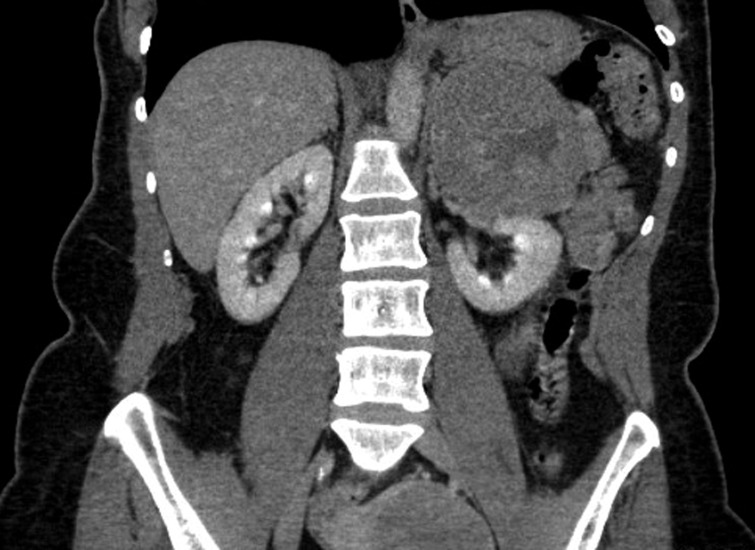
Contrast CT showing a large left-sided adrenal mass with some heterogeneous enhancement and an irregular central cavity probably due to necrosis.
